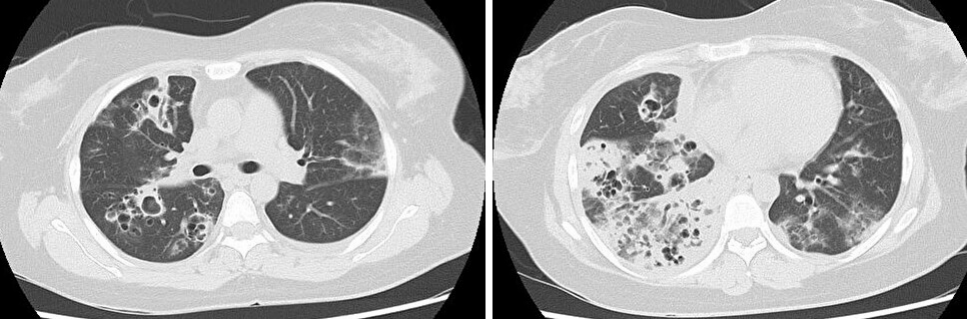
Chest computed tomography obtained on the 10th day of hospitalization, revealing multiple cavitary lesions.
